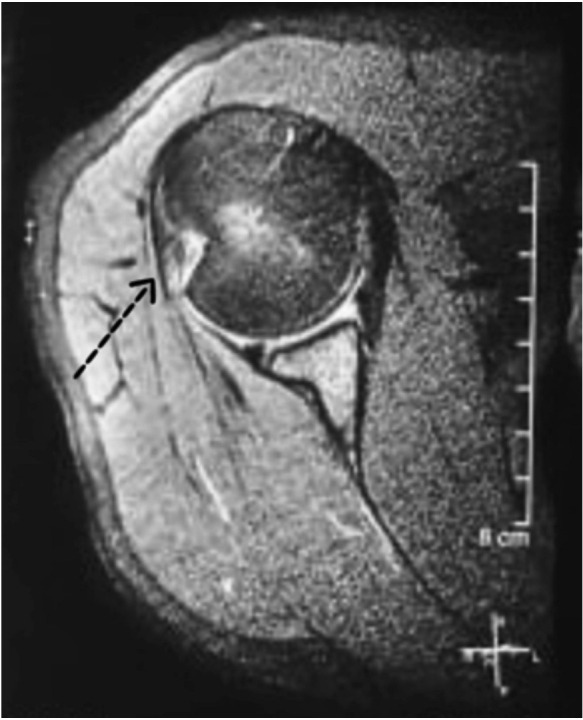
Pre-operative MRI showing Hill Sachs lesion.
